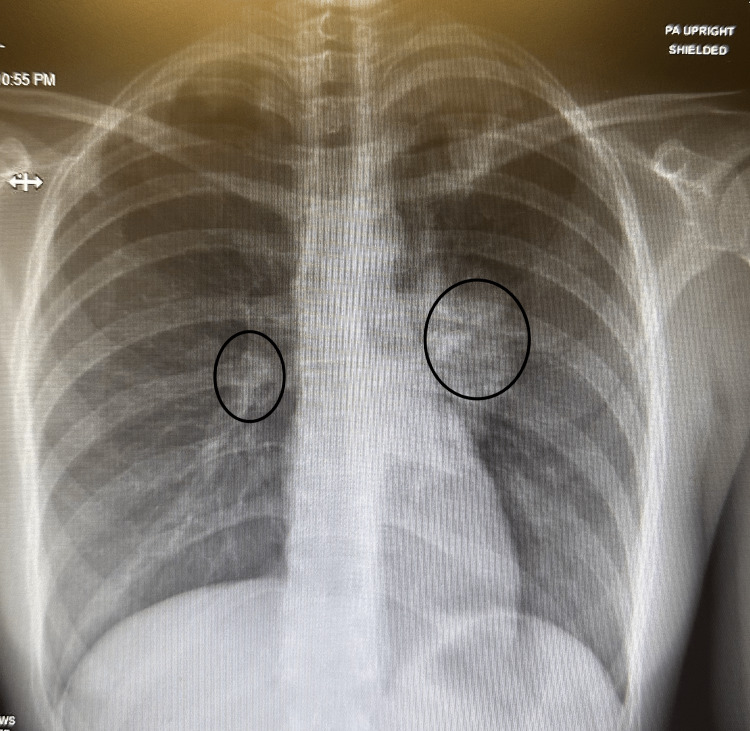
Chest X-rays The circles show mass-like infiltration in the lung parenchyma
